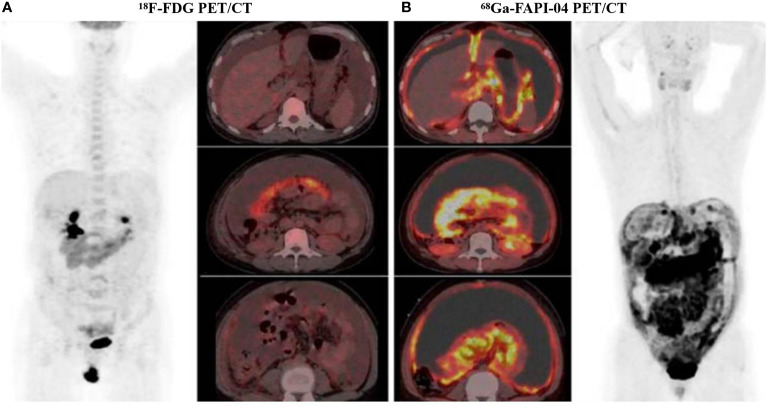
A 47-year-old woman diagnosed with ovarian cancer, ^18^F-FDG PET/CT showed the omental-cake-pattern of peritoneal carcinomatosis with low-to-moderate activity throughout the entire abdomen and pelvis (A). However, there were more details in ^68^Ga-FAPI-04 PET/CT images, we could find that higher uptake was observed in the omental and peritoneal surfaces, especially around the liver and small bowel mesenterium (B). Subsequent pathological findings were consistent with ^68^Ga-FAPI-04 PET/CT findings. ^68^Ga-FAPI-04 PET/CT showed superiority over ^18^F-FDG PET/CT in the display of peritoneal metastatic carcinoma. With permission from Chen et al. (88).
